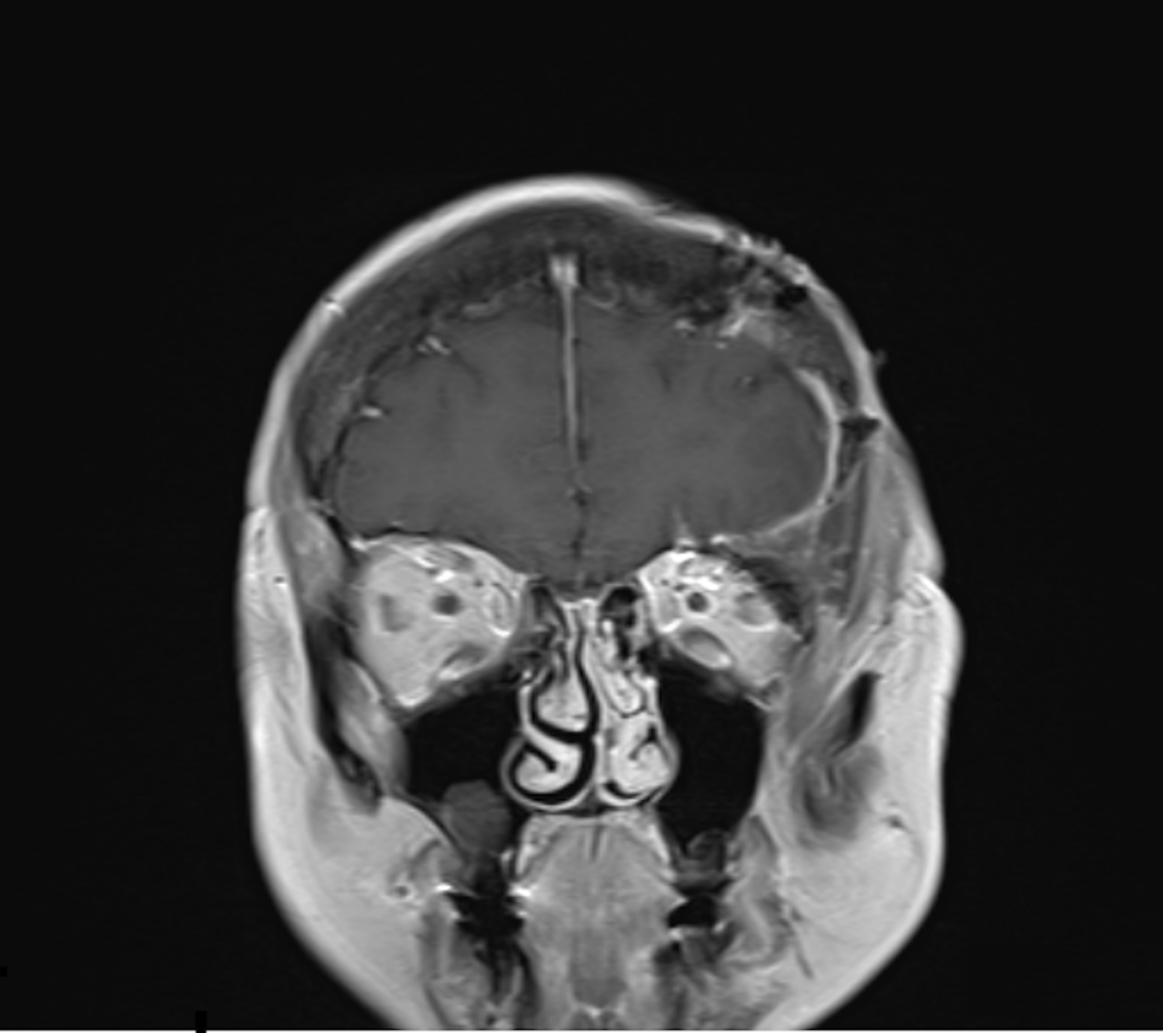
Postoperative coronal T1 contrast-enhanced magnetic resonance imaging demonstrating complete resection of the tumor.
